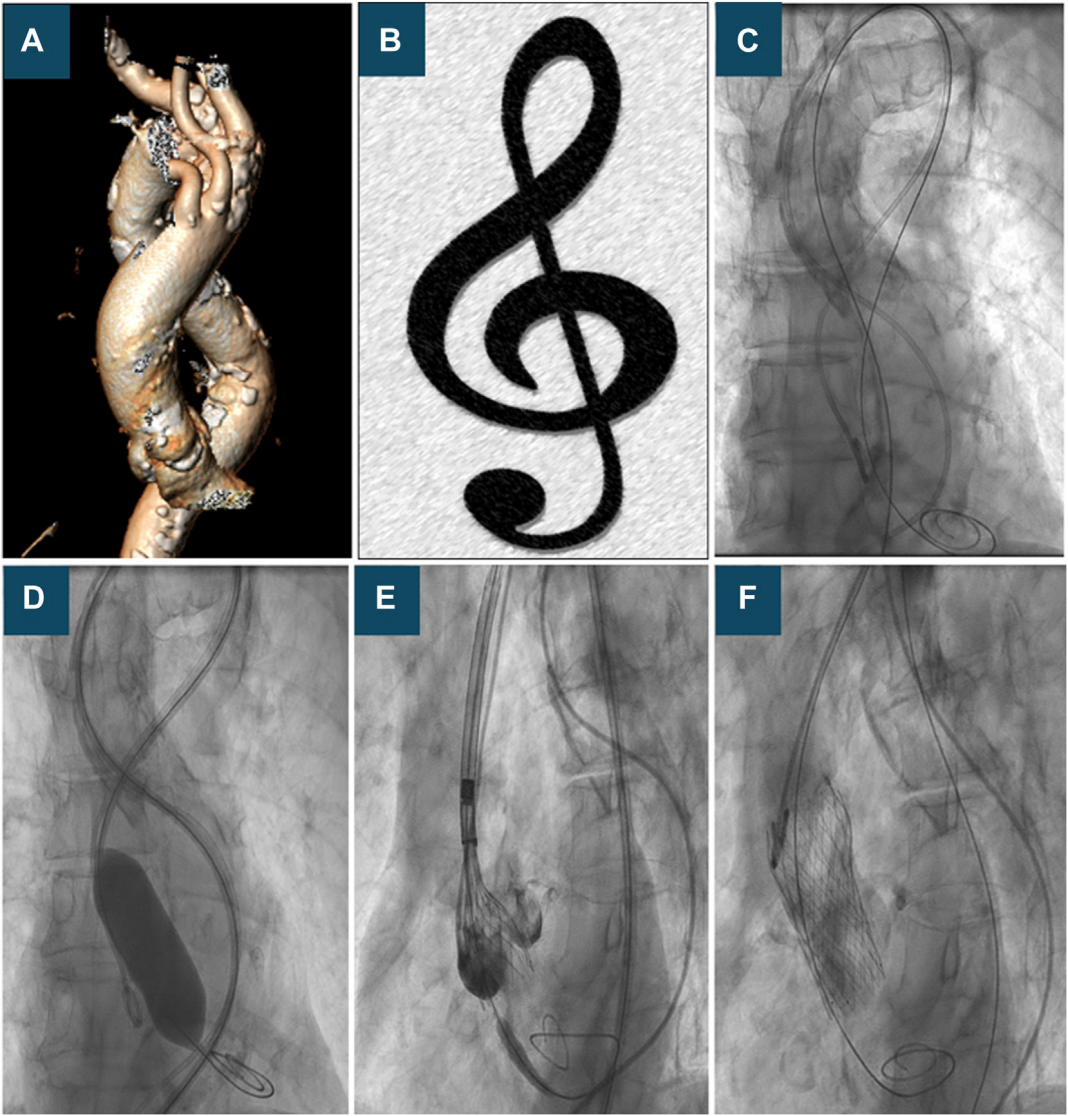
Treble clef aorta: 3D multidetector computed tomography reconstruction and fluoroscopic imaging showed the extremely tortuous and elongated aortic arch resembling the Treble clef musical sign (A-C). Fluoroscopic imaging of transcatheter aortic valve replacement (D-F).
